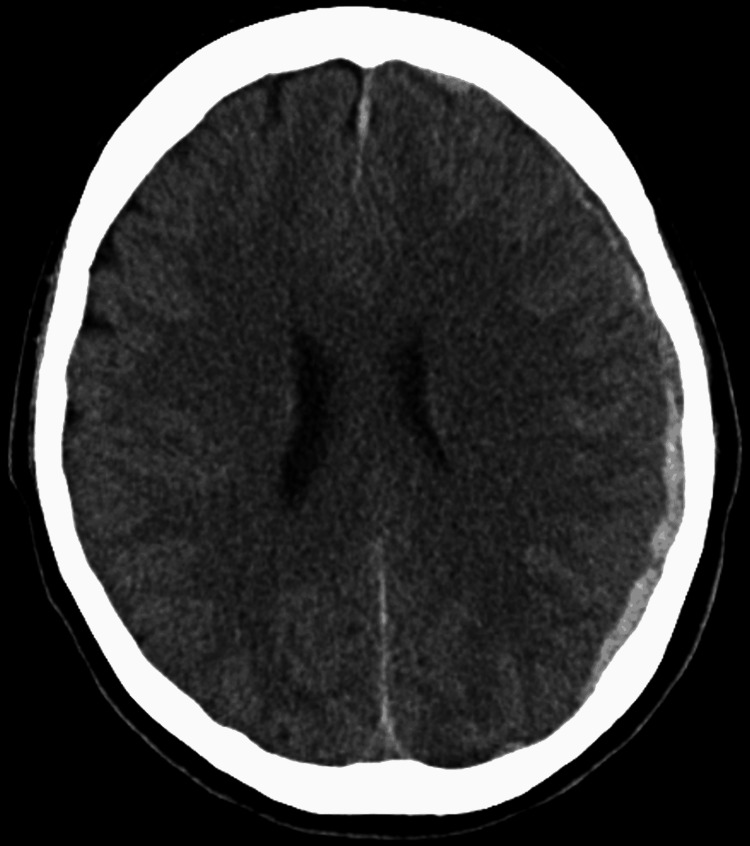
Brain CT at initial examination
